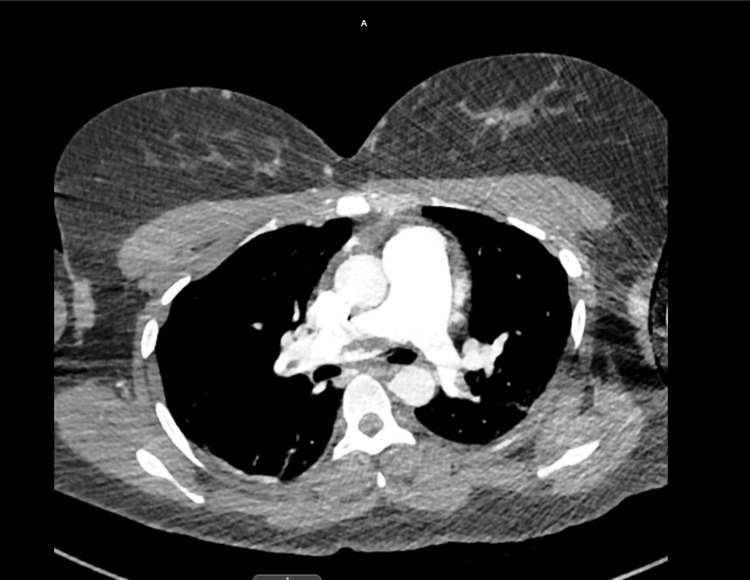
CT thorax with pulmonary embolism protocol showed saddle pulmonary embolism
